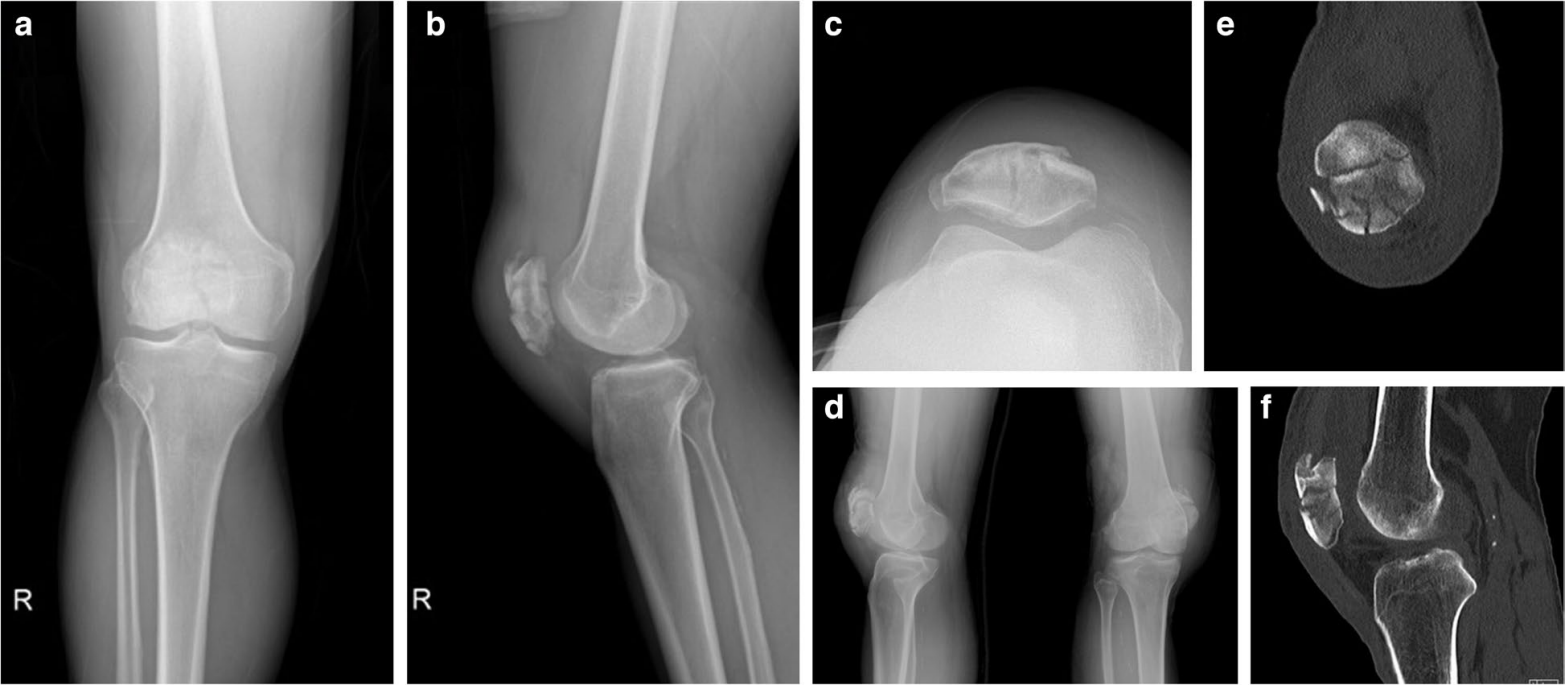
Preoperative radiologic examination of Case 1 (Male, 62 years old) a anterior-to-posterior (AP) view b lateral view c merchant view d both oblique view e computed tomographic (CT) coronal view f CT sagittal view
